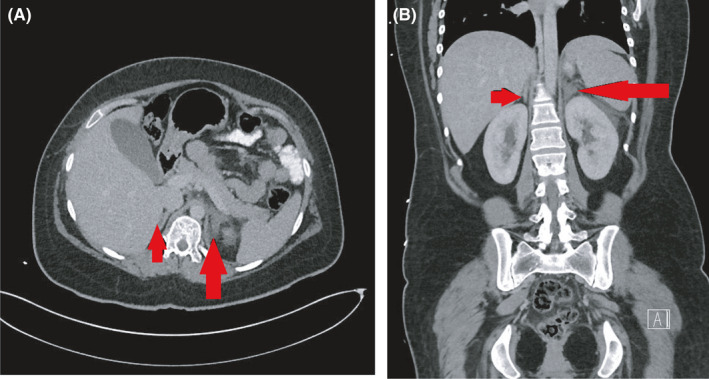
(A) Contrast‐enhanced CT abdomen and pelvis in the axial view. The arrow shows an enlarged left adrenal gland with low attenuation when compared with the right. (B) Contrast‐enhanced CT abdomen and pelvis in the coronal view. The arrow depicts an enlarged left adrenal gland with low attenuation when compared with the right
